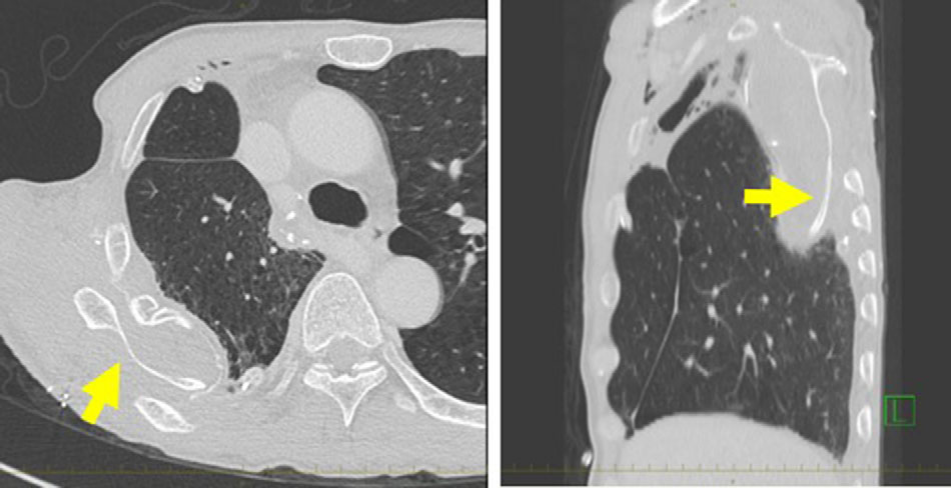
(A) Computed tomography (CT) demonstrating a dislocation of the right scapula in the right thoracic cavity. (B) Sagittal reconstruction of the CT also showed the dislocation of the right scapula.
